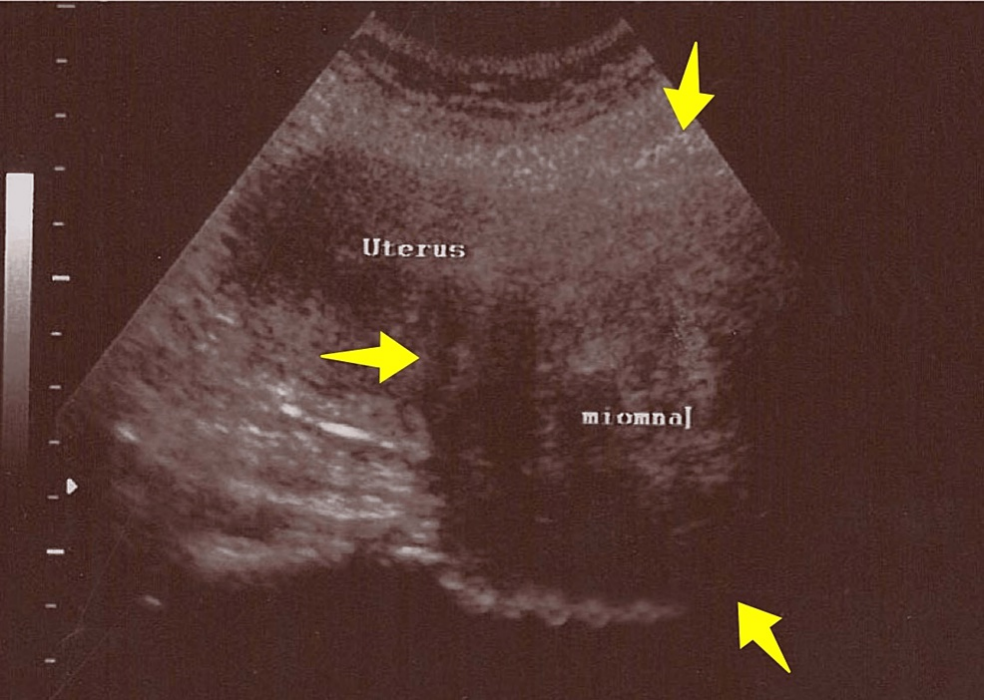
Transabdominal ultrasound imaging of uterine cervical leiomyoma (our case) In the anatomical position of the cervix, the demarcation of a space-occupying lesion (yellow arrows), several times the size of the uterine corpus, is evident.
